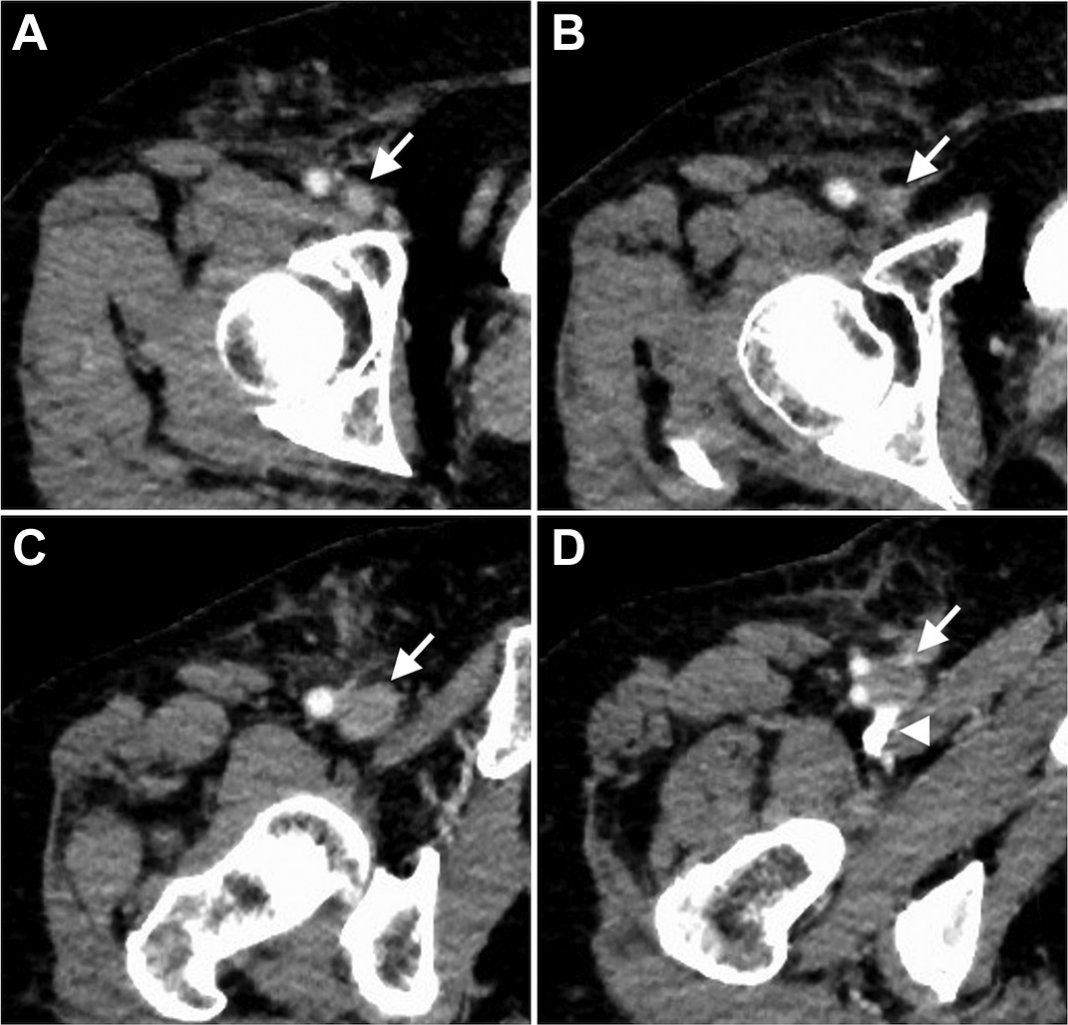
Contrast-Enhanced Computed Tomography of the Right Femoral Vein Occlusion The right femoral vein (arrow) is shown in a proximal (A) to distal direction (D). Contrast enhancement is observed in the proximal side (A), but it is unclear close to the puncture site (B). The distal side is dilated (C) and contrast retention is observed (D, arrowhead).
